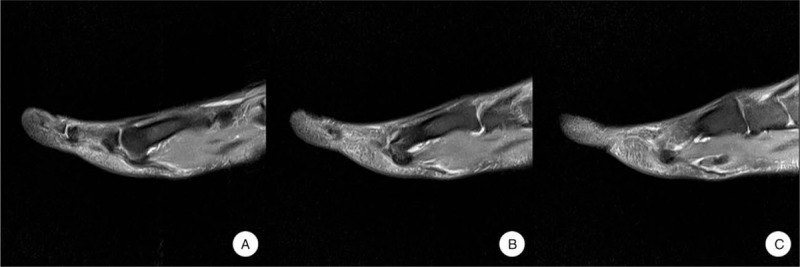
Preoperative magnetic resonance images show that the FHL tendon was completely ruptured at the metatarsal head and neck region and displaced. FHL = flexor halluces longus.
